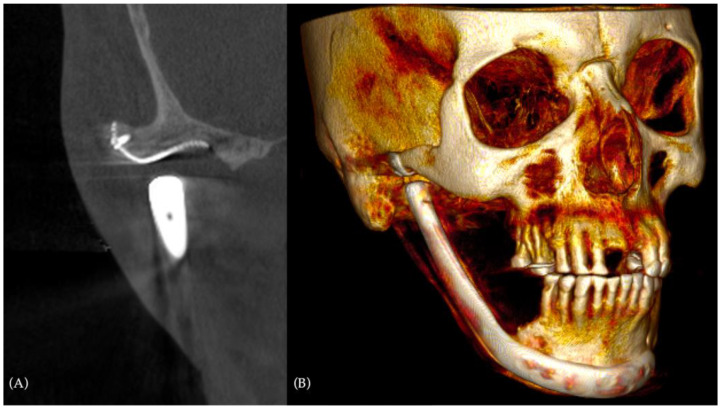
The 3-year-follow-up CT scan: (A) detail of the appropriate fitting of the alloplastic fossa to the temporal bone in coronal view; (B) three-quarter right view of the CT scan 3D reconstruction, showing stability of the obtained result.
